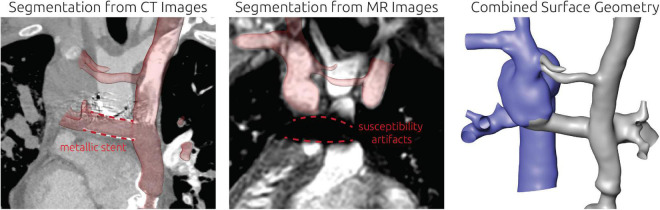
Detailed overview of the segmentation strategy for the second patient. The left and mid panel show the segmentation from CT and CMR images respectively. The right panel shows the final surface geometry that was reconstructed by combination of both segmentations. Tissue highlighted in blue was reconstructed from CMR images, whereas the remaining tissue was reconstructed from CT images. Additionally, the location of a metallic stent in the TCPC is highlighted in the CT images together with the resulting magnetic susceptibility artifacts during CMR imaging.
